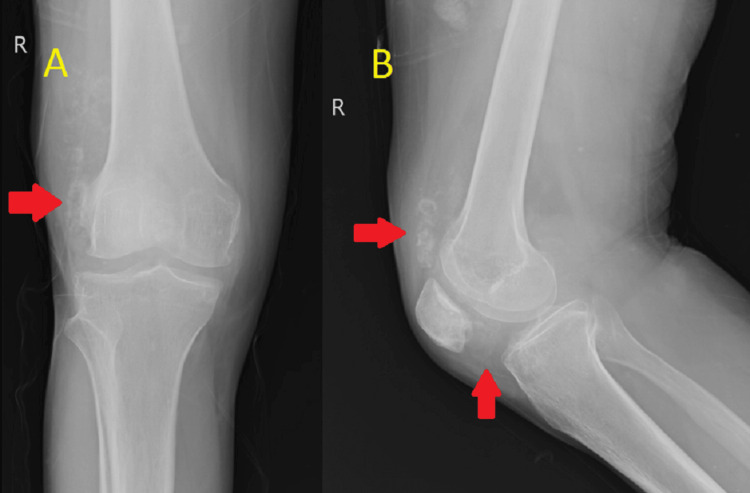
Anteroposterior (A) and lateral (B) radiographs of the knee showing multiple lesions like loose bodies Image A shows loose bodies as depicted by the arrow on the lateral aspect of the knee. Image B shows loose bodies in the suprapatellar and infrapatellar region
